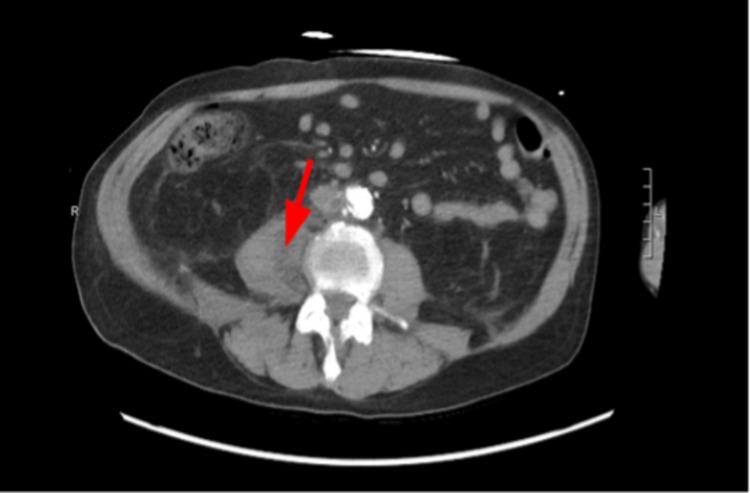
Initial CT showing psoas abscess (red arrow).
